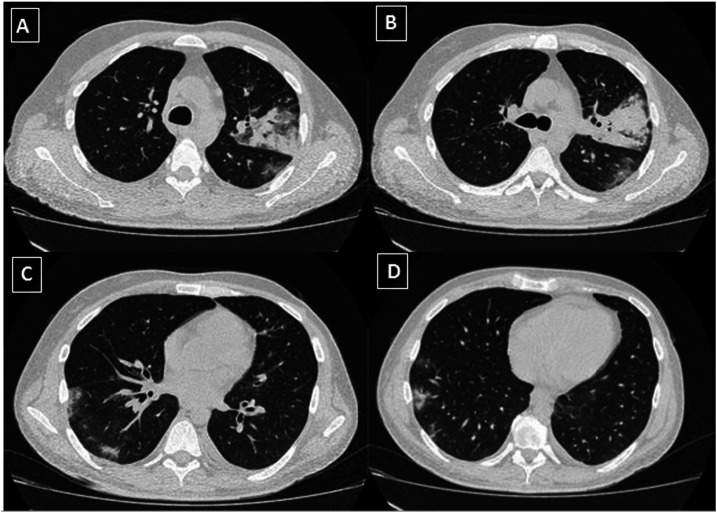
Chest computed tomography (CT). (A and B) Axial thin-section unenhanced CT image revealing consolidative pulmonary opacities in the left upper lobe. (C and D) Axial thin-section unenhanced CT image showing multifocal, rounded, and bilateral ground-glass opacities, with peripheral and posterior predominance in the right lower lobe.
